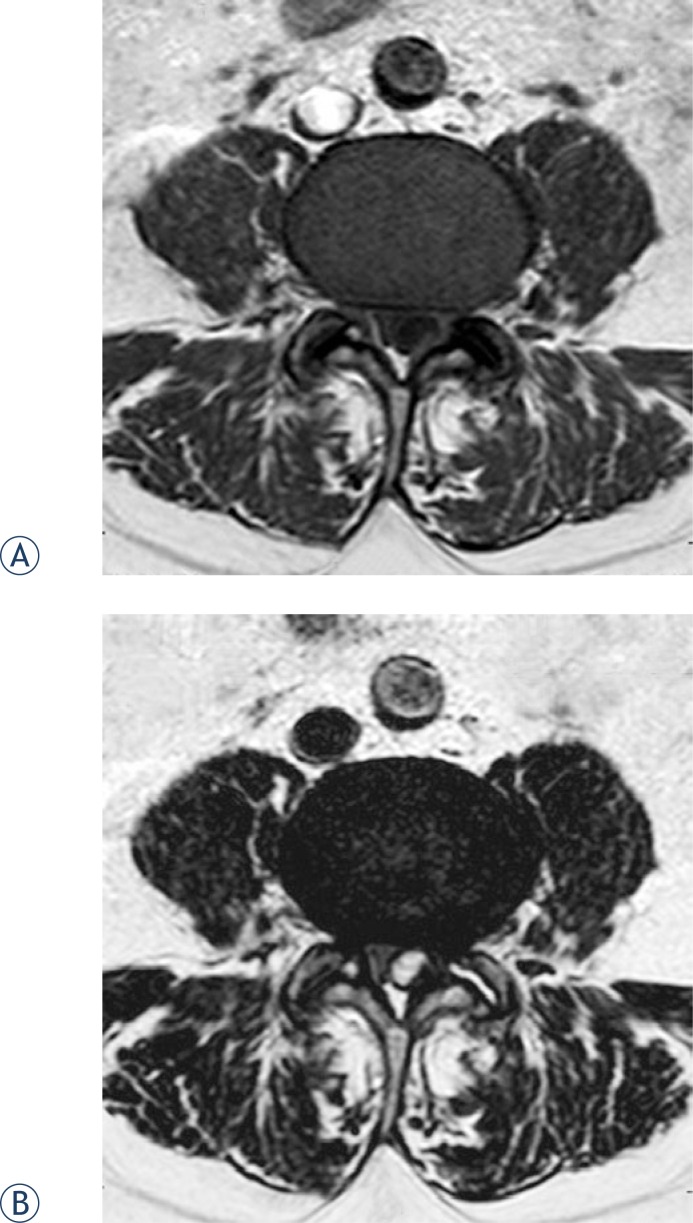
Baseline (preoprocedural) MRI in an axial plane at the level of the L4–L5 lumbar segment. Hypointense well delineated cystic formation compressing dural sac from the left dorsolateral side is seen on T1 SE image (A). The cyst that is hyperintense on T2 FSE image shows continuation with the left facet joint (some fluid can also be seen in the degenerated facet joint) (B).
